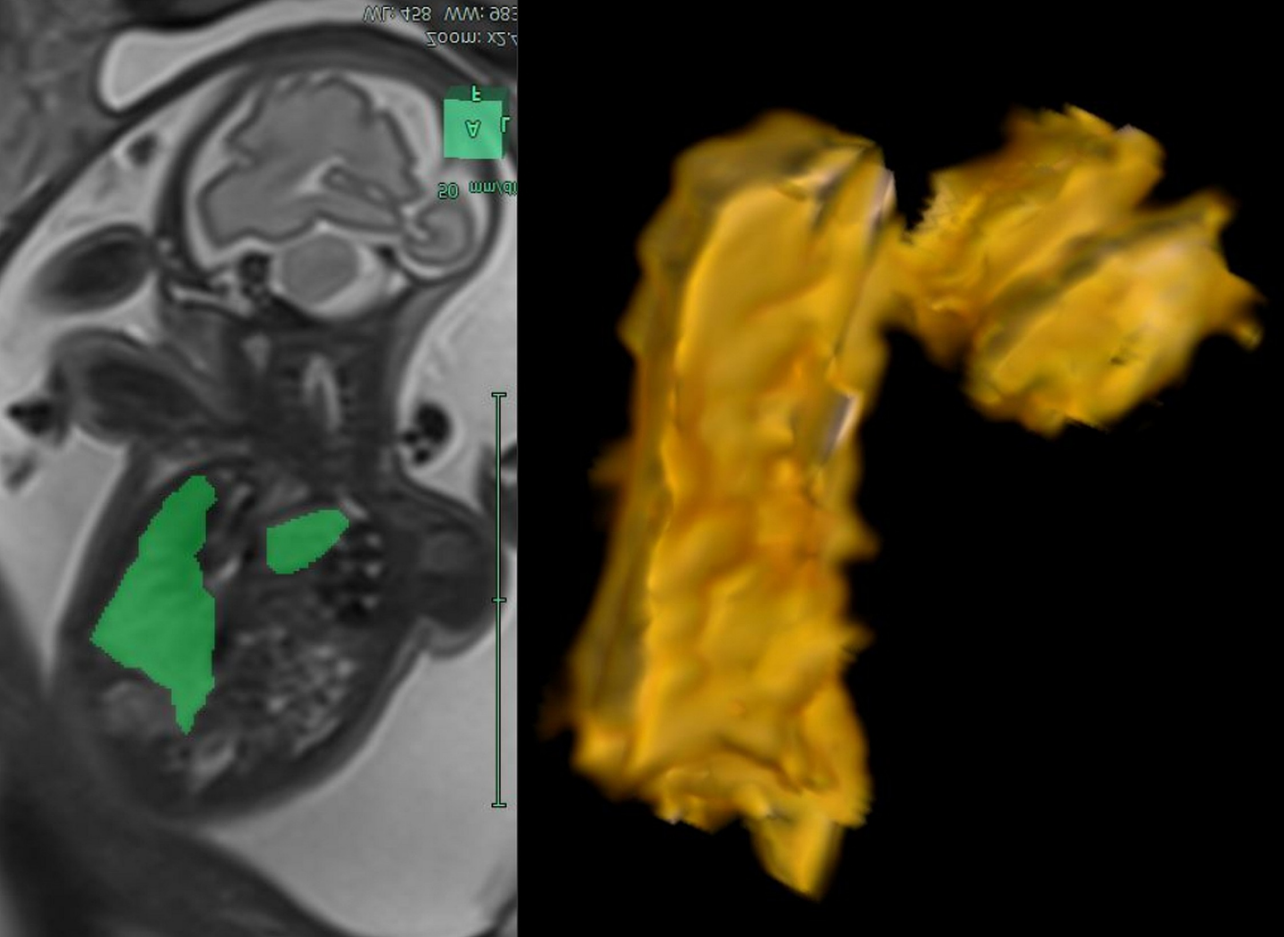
Total fetal lung volume assessment. MRI segmentation and 3D reconstruction of the fetal lung with the calculation of the total fetal lung volume on T2-sequences.
